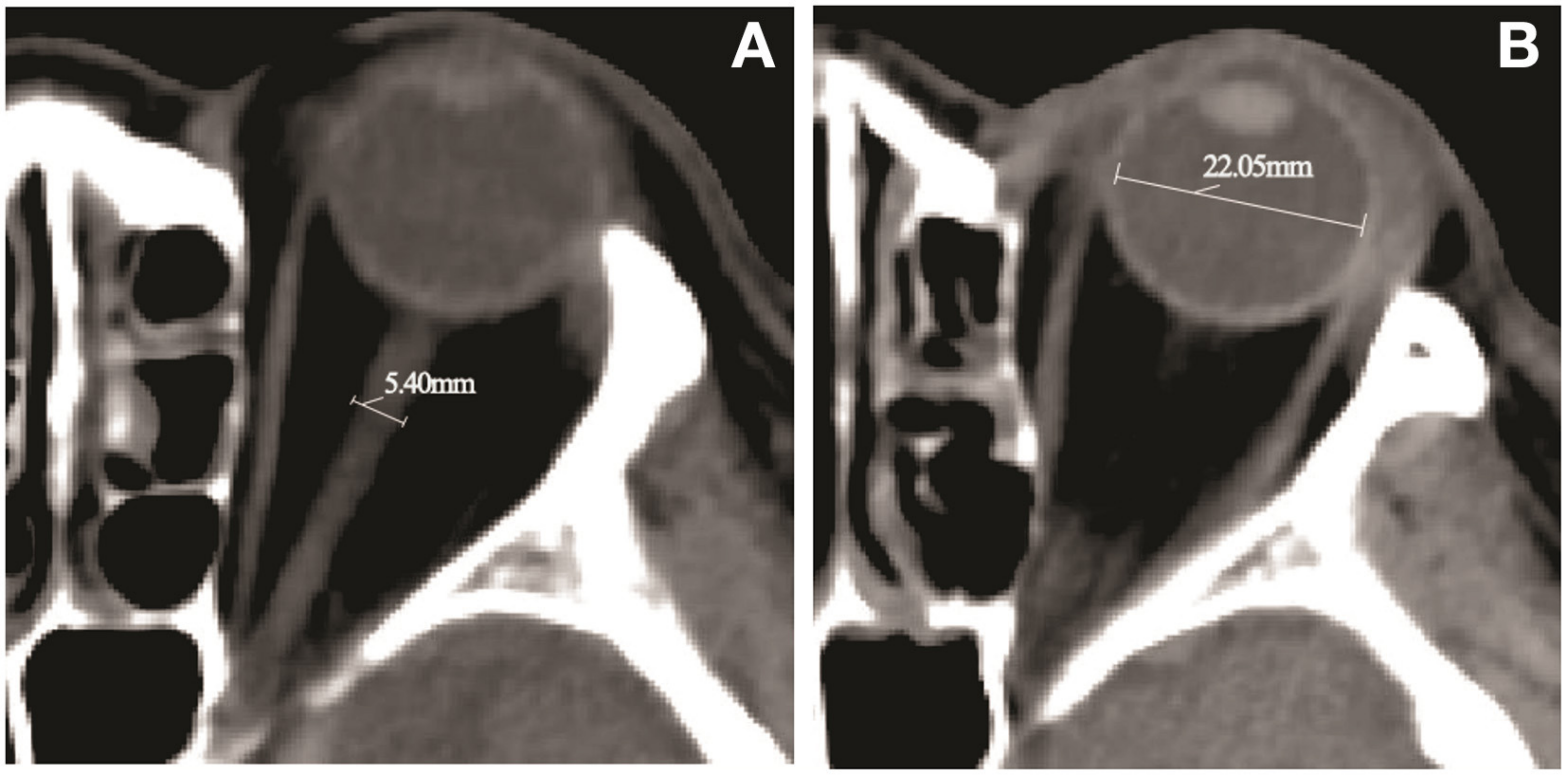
Computed tomography (CT) image of a 69-year-old female patient with a middle cerebral artery (MCA) infarction due to an occlusion of the proximal M1 segment. (A) The measurement of the optic nerve sheath diameter (ONSD) indicates diameter of 5.40 mm. (B) The eyeball transverse diameter (ETD) retina to retina measurement by head CT scan indicates diameter of 22.05 mm.
